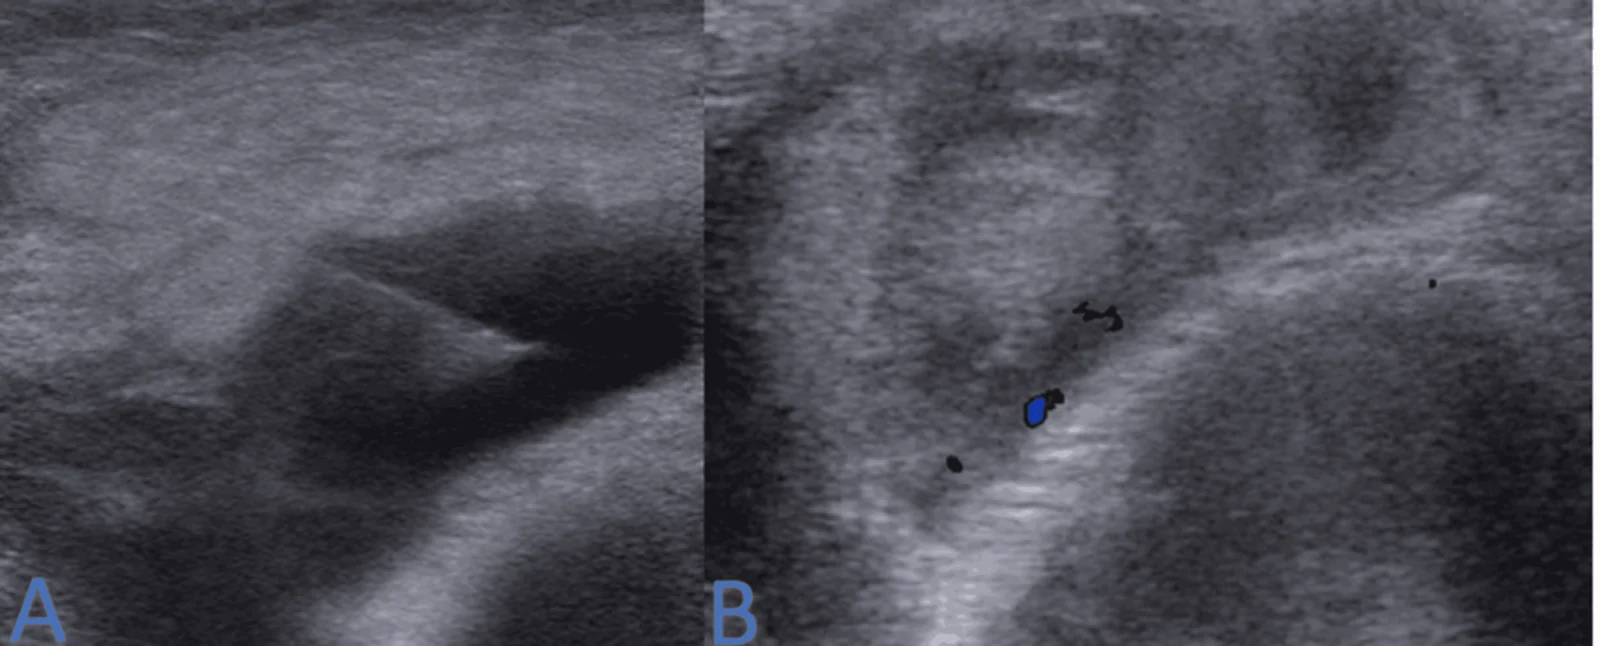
Ultrasound images of the knee (A), (B) Transverse ultrasound images showing a 22-gauge needle inserted into the genicular artery pseudoaneurysm with thrombin injection.
